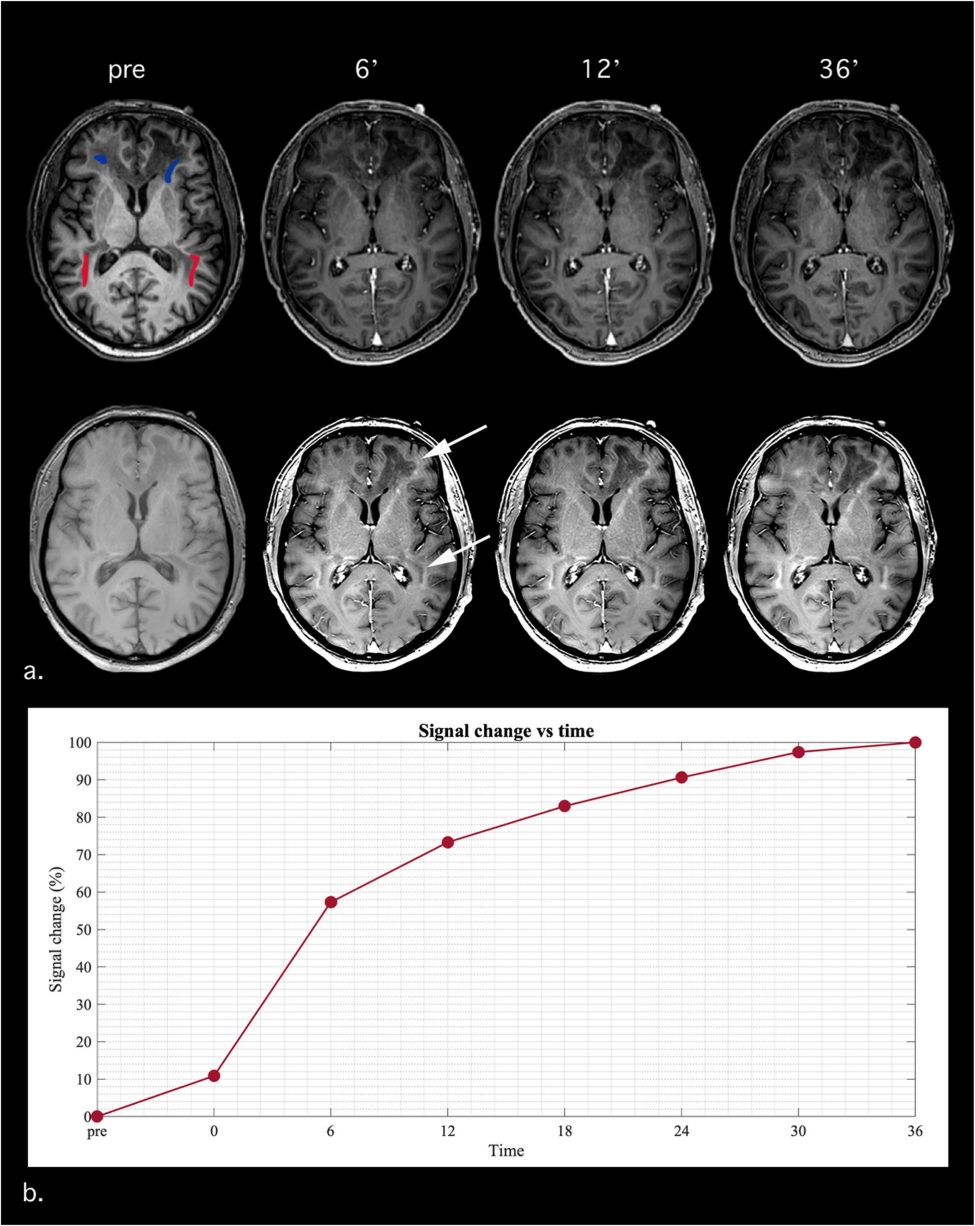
Variation of contrast enhancement detection on different T1-weighted sequences at different time points in the same adrenoleukodystrophy patient shown in Fig. 1. a, top row: no contrast enhancement is visible on the T1-weighted three-dimensional (3D) gradient-echo sequence before and 6, 12, and 36 min after gadobutrol administration. a, bottom row: the T1-weighted two-dimensional (2D) gradient-echo sequence before and 6, 12, and 36 min after gadobutrol administration show the presence of contrast enhancement from 6 minutes on; the arrows indicate the areas of enhancement on the 6-min image. b Signal intensity variation, expressed as percentage of its maximum value, is plotted against time for the T1-weighted 2D gradient-echo sequence as average of the two regions of interest (ROIs) drawn on the precontrast image of the 3D series (one ROI in the frontal lobes, considering two blue areas; one ROI in the parietal lobes, considering two red areas) to allow a clean evaluation of the 2D series
